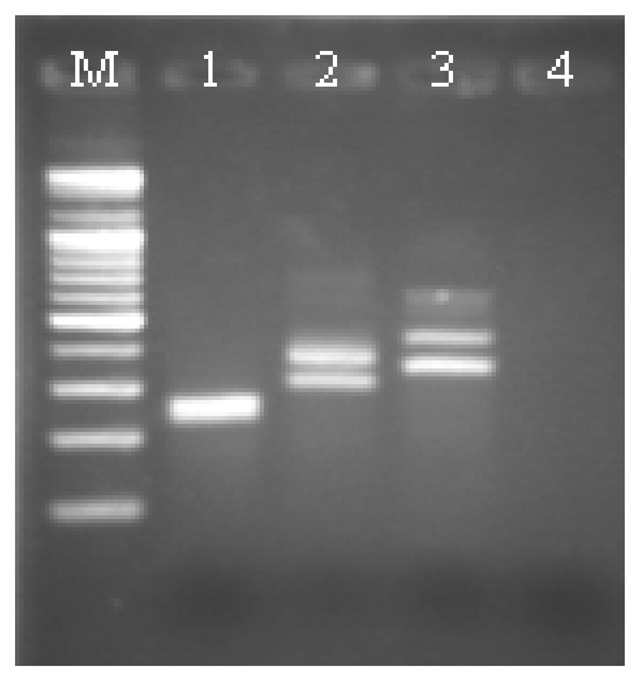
The corresponding gel electrophoresis of the PCR analytes for the selectivity tests. Channels M: the ladder DNA marker; 1: *Arabidopsis thaliana* (252 b); 2: H5 AIV (358 b) 3: PSMA5 (432 b); and 4: negative control. Double bands on each channel indicated the presence of both single and double stranded DNA analytes in the PCR products. *Arabidopsis thaliana*'s on Channel 1 obviously was too short in size to display discrete bands on this gel.
